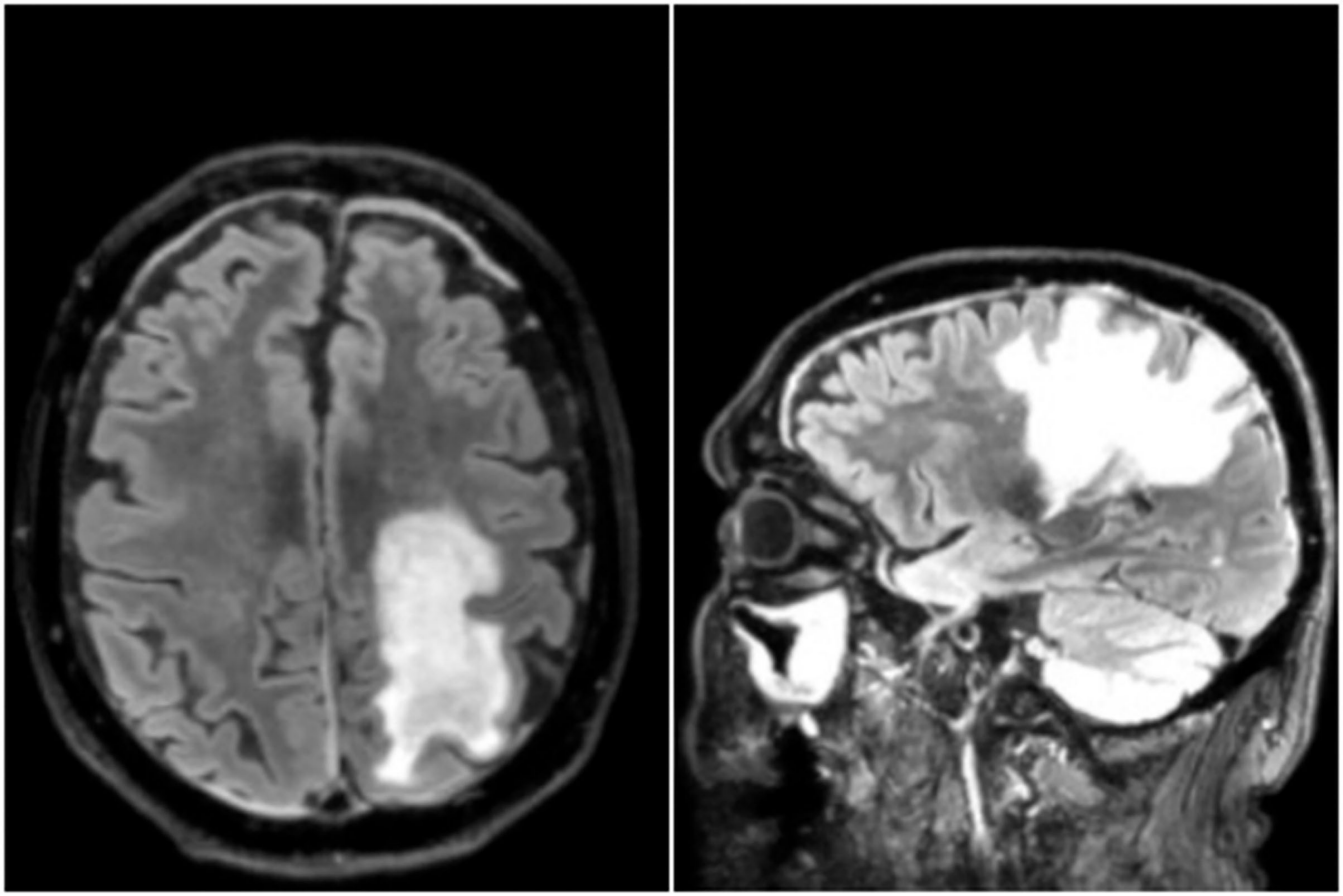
Axial (left) and sagittal (right) T2-FLAIR MRI showing a white matter hyperintensity in the left fronto-parietal region.
